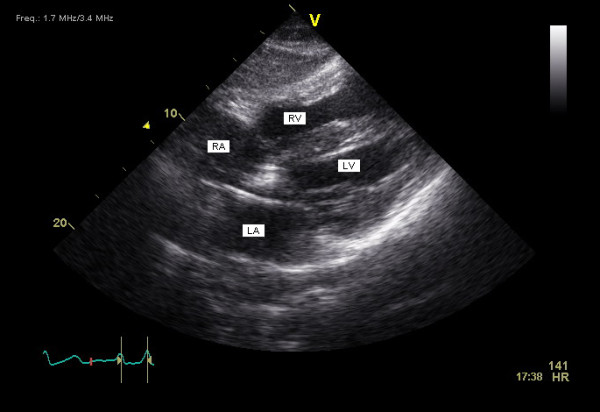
Transthoracic echocardiography, subcostal long axis view showing the pacing lead to pass from the right atrium via the patent foramen ovale to the left atrium, then via the mitral valve to the left ventricle. RA, right atrium; LA, left atrium; RV, right ventricle; LV, left ventricle.
